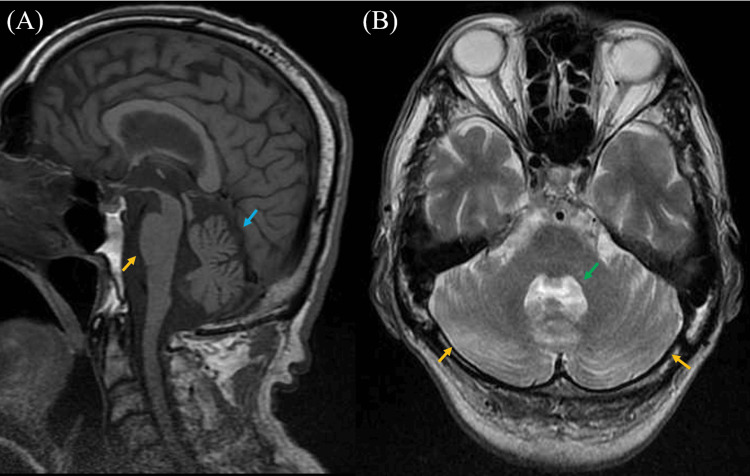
Results of brain MRI (A) T1-weighted brain MRI showing atrophy of the basilar pons (yellow arrow) and cerebellum (blue arrow). (B) T2-weighted brain MRI showing atrophy of the cerebellum (yellow arrows) and enlargement of the fourth ventricle (green arrow). MRI: Magnetic resonance imaging
